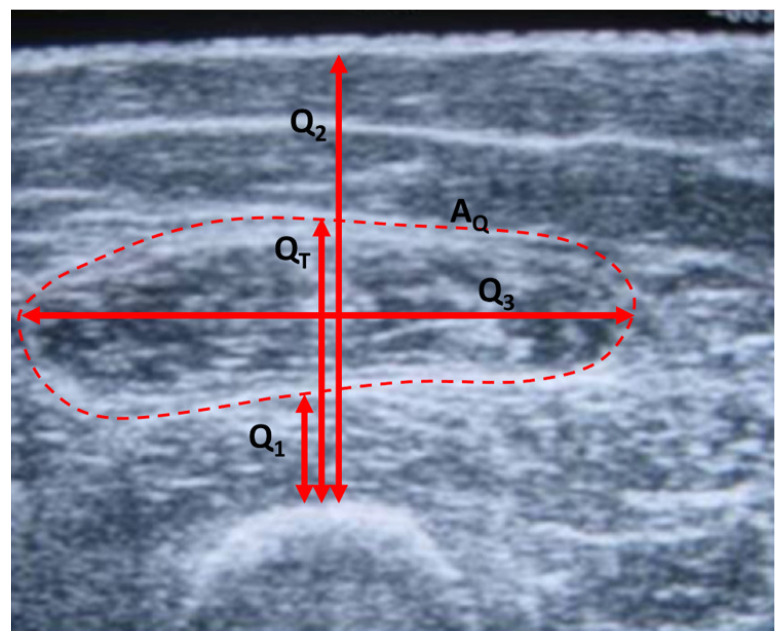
Thickness $Q_{T}$ and the area $A_{Q}$ on an ultrasound image of the quadriceps.
